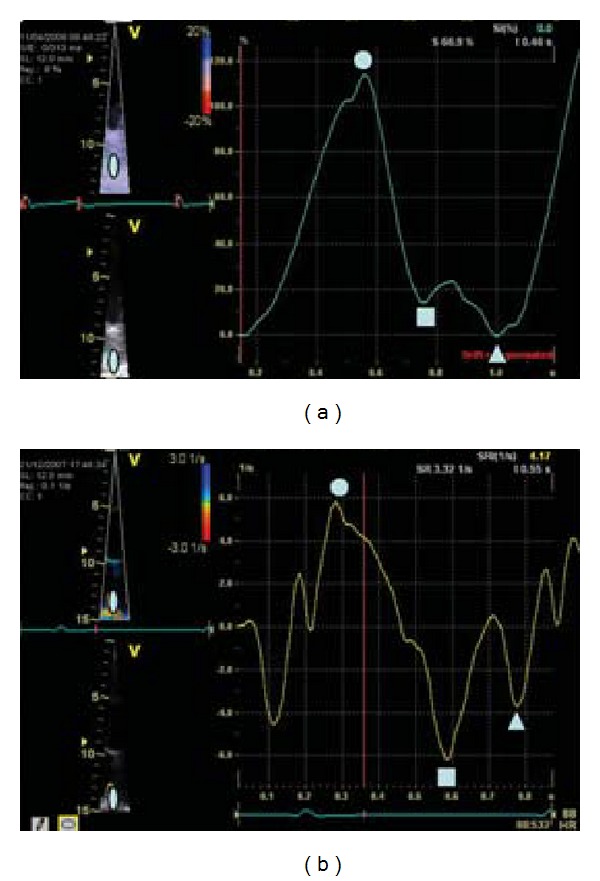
Left atrial strain (a) and strain rate (b) curves by Doppler. Left atrial reservoir function is studied by peak systolic value (ball), left atrial conduit function by peak early diastolic value (square), and left atrial pump function by peak late diastolic value (triangle).
